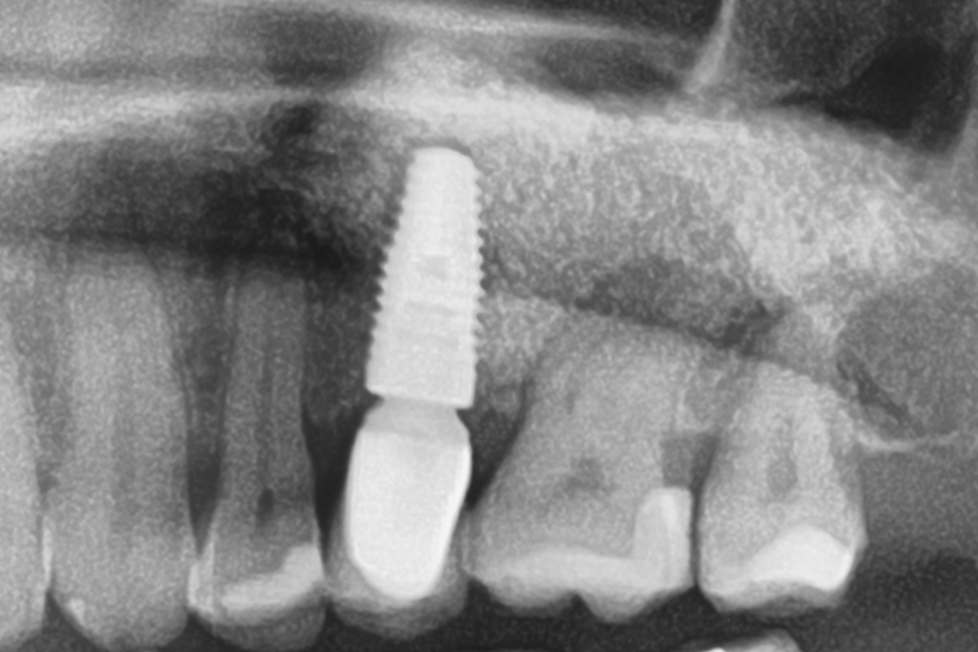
Follow-up radiograph after one year of healing
